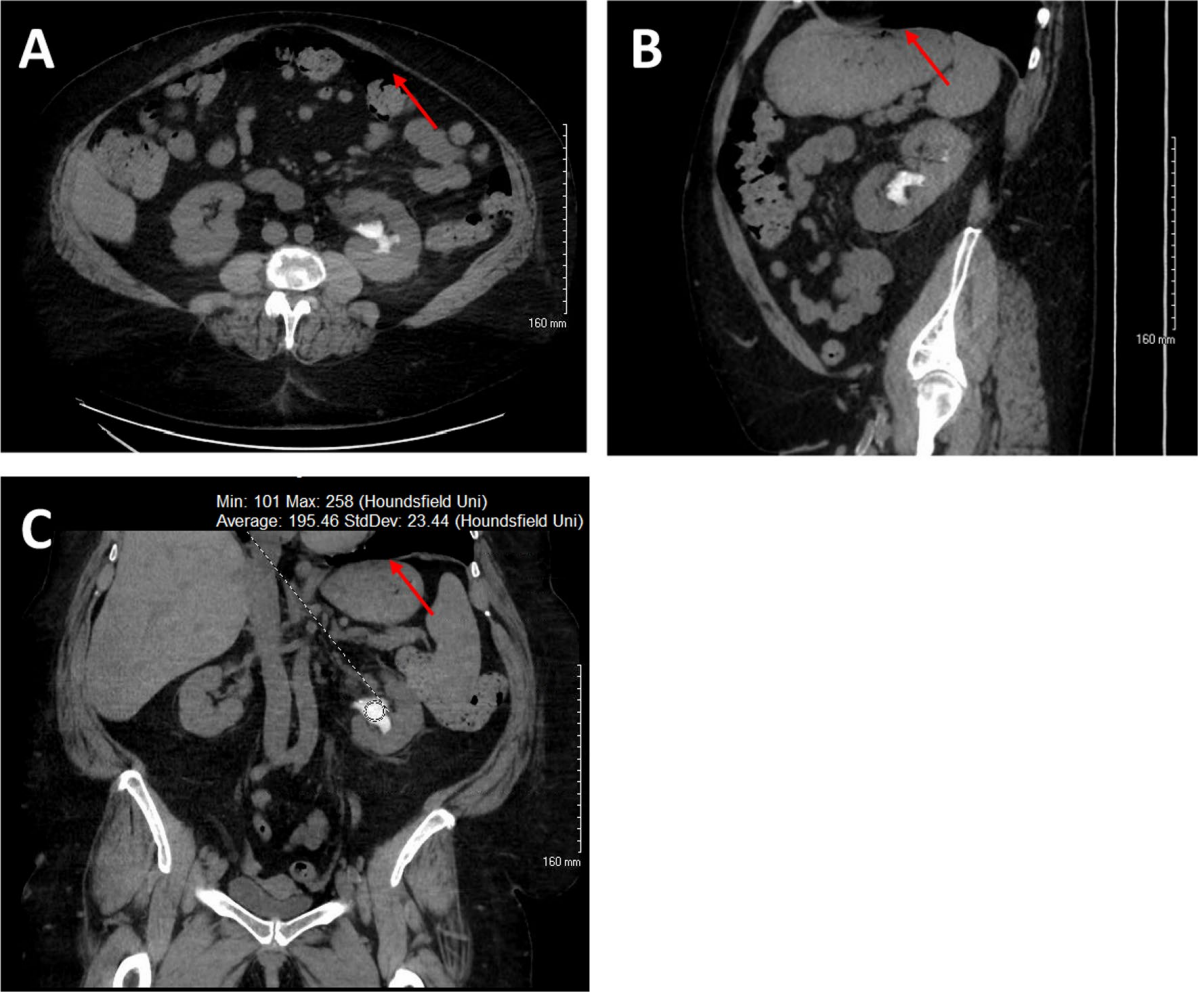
Radiographic images of left renal pelvis stone (red arrow) in the axial (a), sagittal (b), and coronal (c) on non-contrast CT abdomen and pelvis. The stone was demonstrated on imaging to have a density of 194.46 Hounsfield Units
